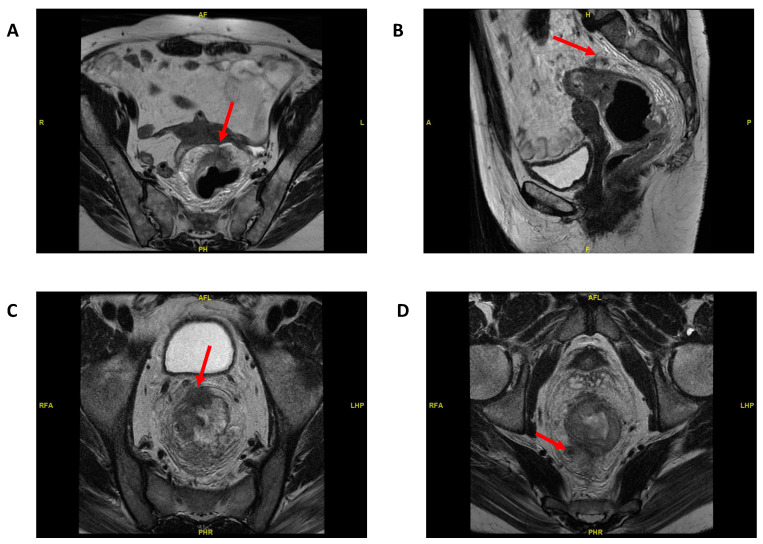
MRI from patients showing locally advanced rectal cancer with high-risk features. (A) Upper third rectal cancer with peritoneal reflection invasion (cT4a). (B) Same patient showing extra-mural vascular invasion. (C) Lower third rectal cancer in a male with invasion of the anterior part of the mesorectal fascia (cT3d) and multiple large size peritumoral lymph nodes (N2) (D).
